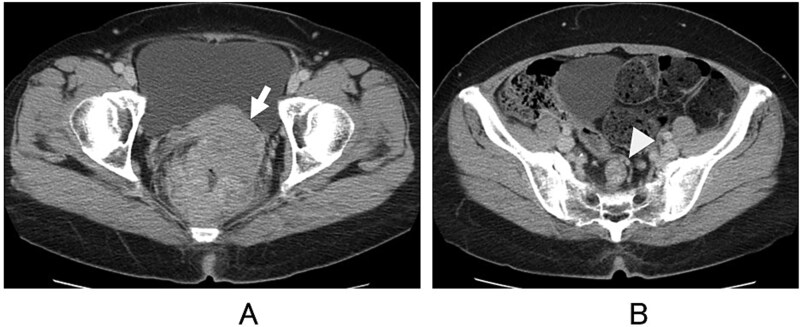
Computed tomography. Direct invasion of the uterus (A, arrow) and regional lymph node metastasis (B, arrowhead) are shown.
